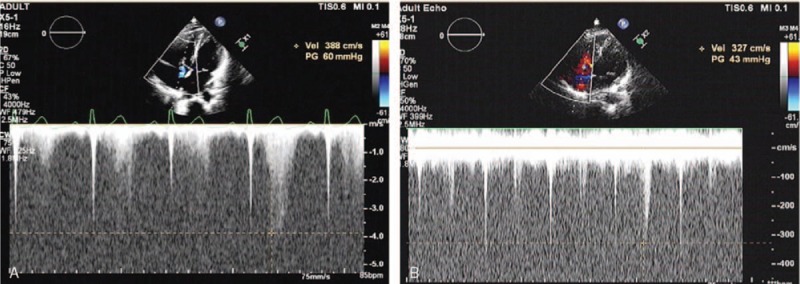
Echocardiography with the tricuspid insufficiency method showed that the systolic blood pressure of the pulmonary artery was ∼70 mm Hg in April 2015 (A) and May 2016 (B). CT = computed tomography.
